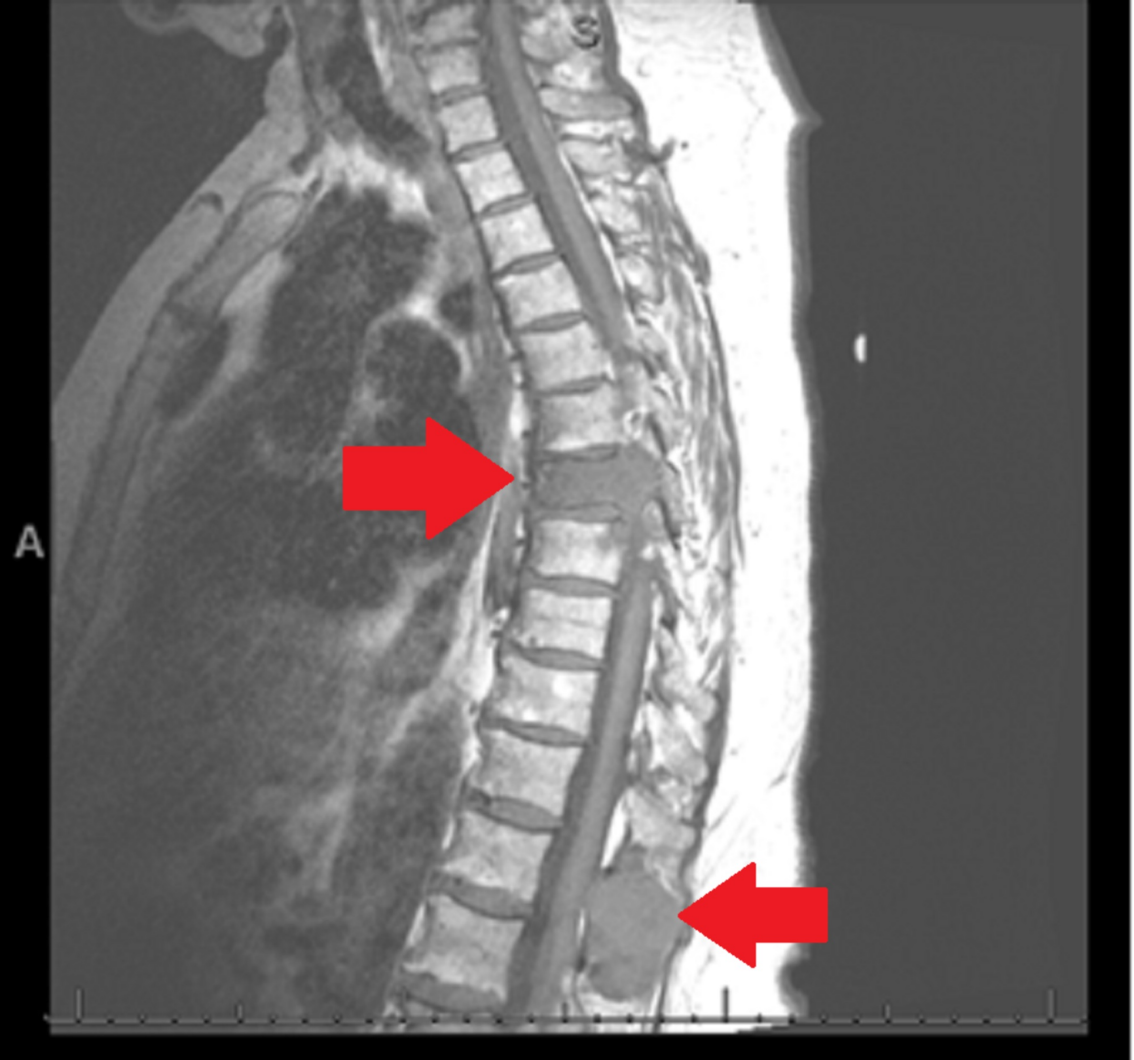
Lesions of T7 and T12 via magnetic resonance imaging T1 weighted image of thoracic spine demonstrating pathologic lesions of T7 and T12
